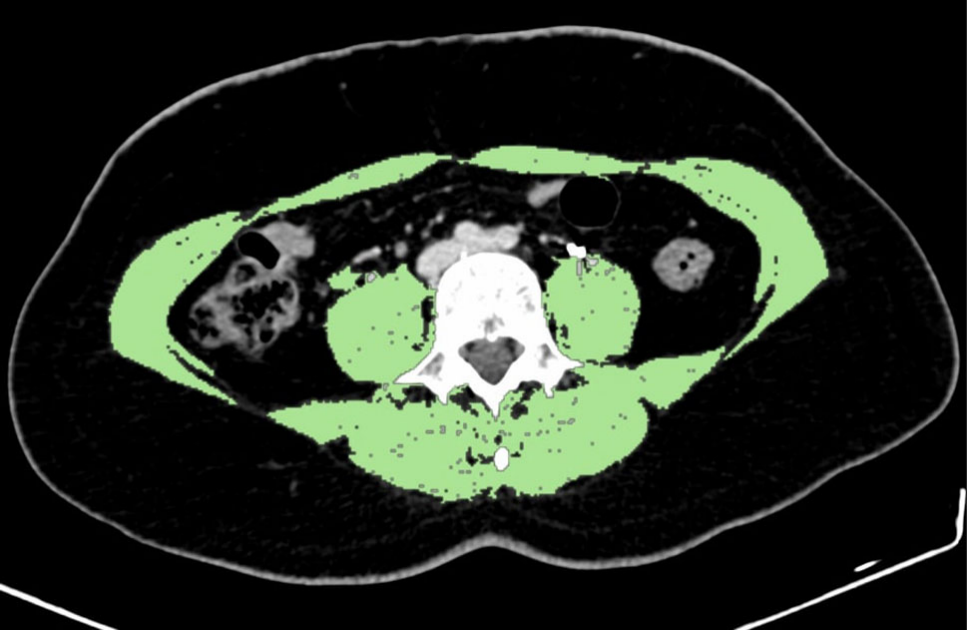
Axial CT image at the L3 vertebral level showing segmentation of abdominal skeletal muscles
